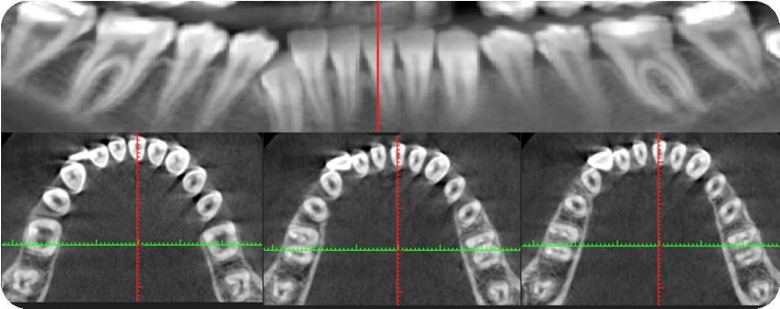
CBCT image and its different cross sections of bilateral mandibular second molars
